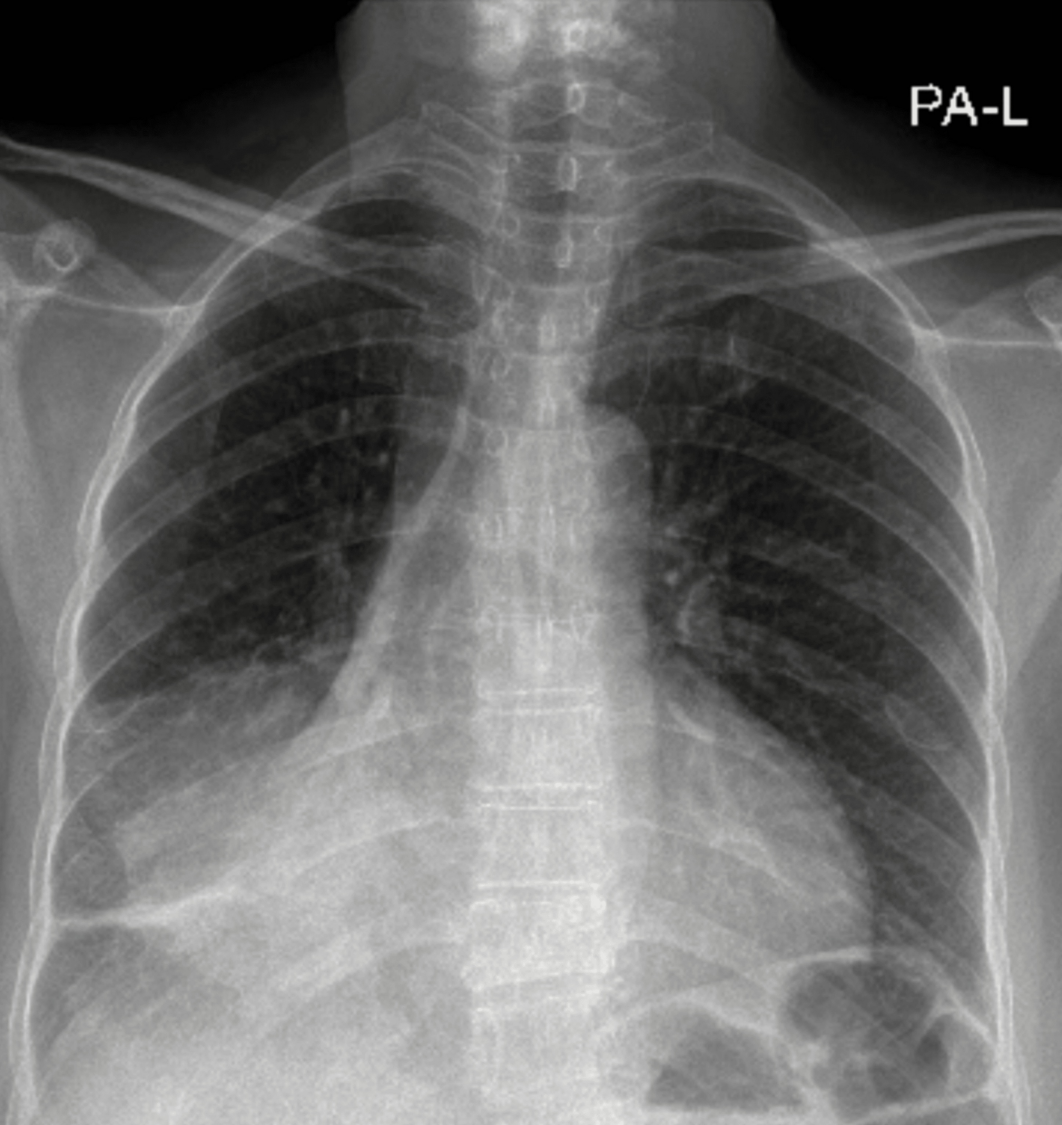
The chest radiography shows a mild increase in cardiac silhouette, tracheal deviation to the right side, and opacification in the right lower zone of the lung, suggestive of a mass lesion with lower lobe collapse.
